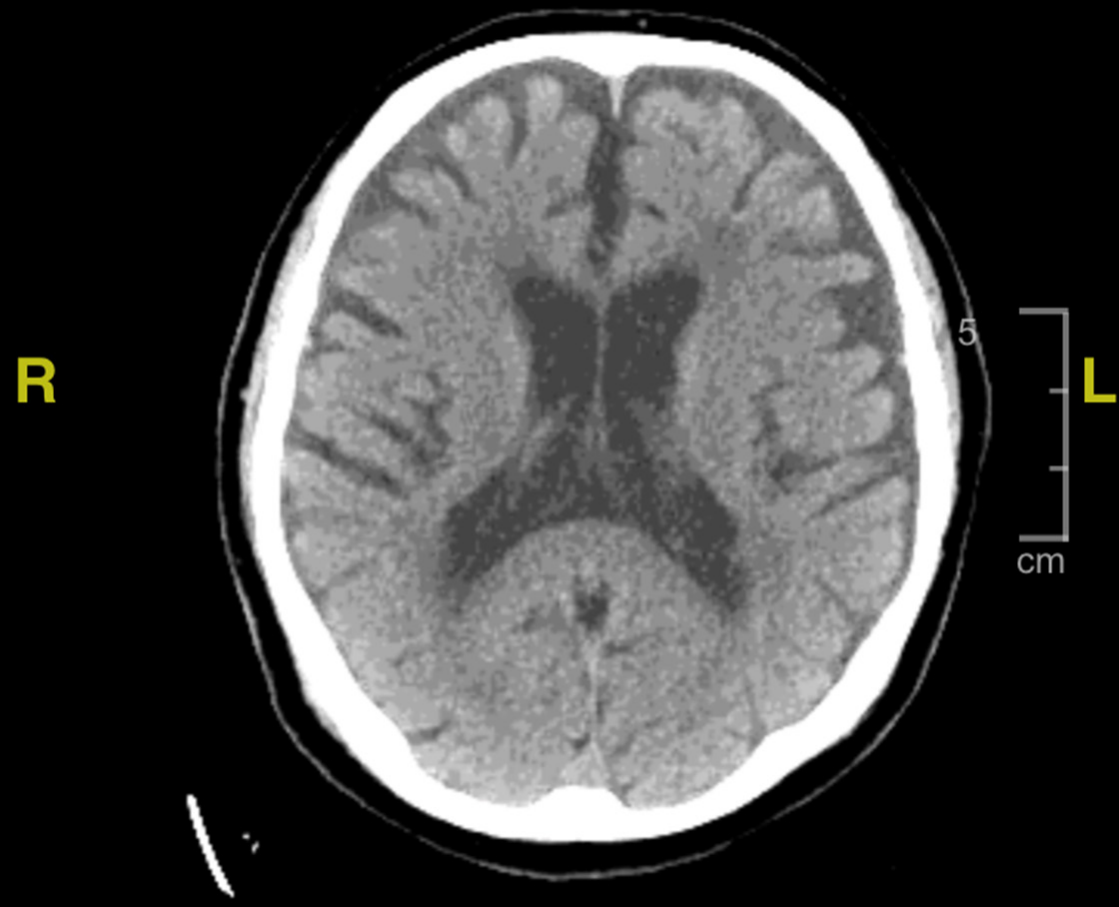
Brain CT showing no acute intracranial pathology.
